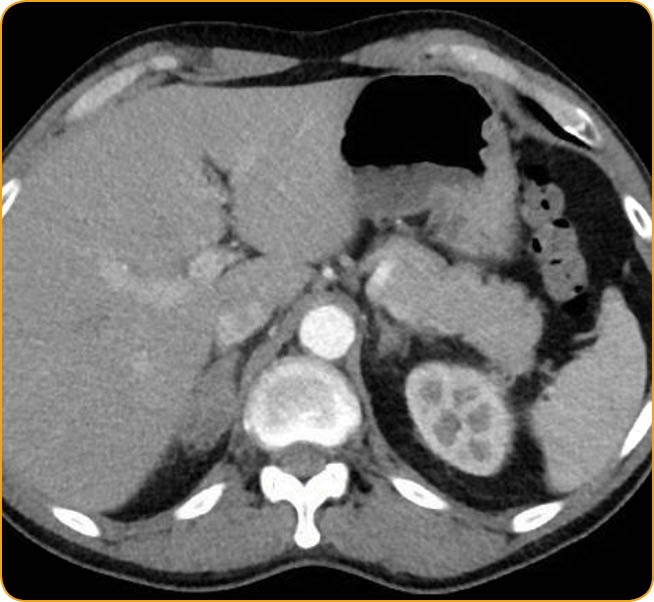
CT image shows the liver, which appears to be clear, but the adrenal gland is thickened and enlarged on the right side.
